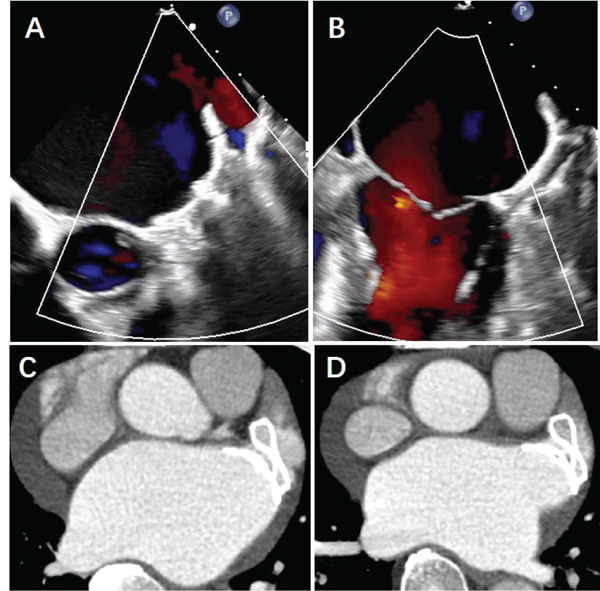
TEE and CT angiography images of the split occluder after implantation in human. (A–D) TEE and computed tomography (CT) images at 90 days after implantation of the combination of the fixed and covering discs. (A–B) TEE revealed that the occluder was stable and exhibited no evident PDL or device‐related thrombosis. (C–D) CT angiography revealed that the occluder was stable and exhibited no device‐related thrombosis but a minor PDL.
